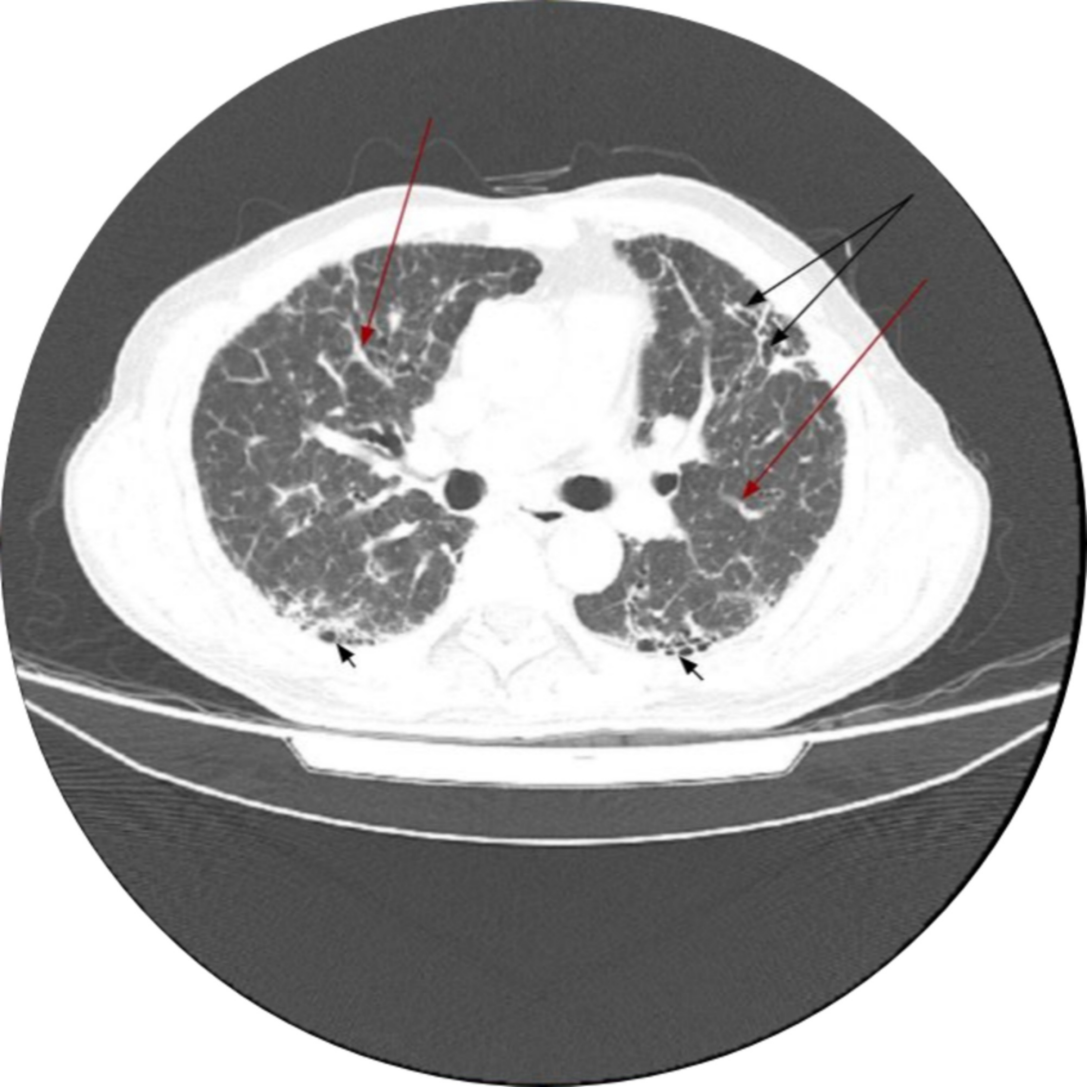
High-resolution computed tomographic features of usual interstitial pneumonia Diffuse interlobar interstitial thickening is noted at bilateral aerated lungs (*red arrows*) along with honeycombing more prevalent at lung bases (*short black arrows*) and mild traction bronchiectasis at left upper lobe (*longer black arrows at the top*)
